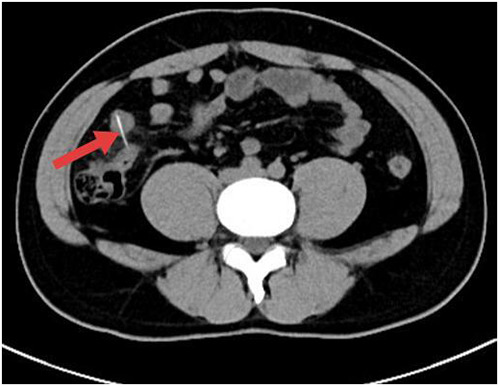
CT scan showing a linear hyperdense foreign body (red arrow) in the right lower quadrant.
